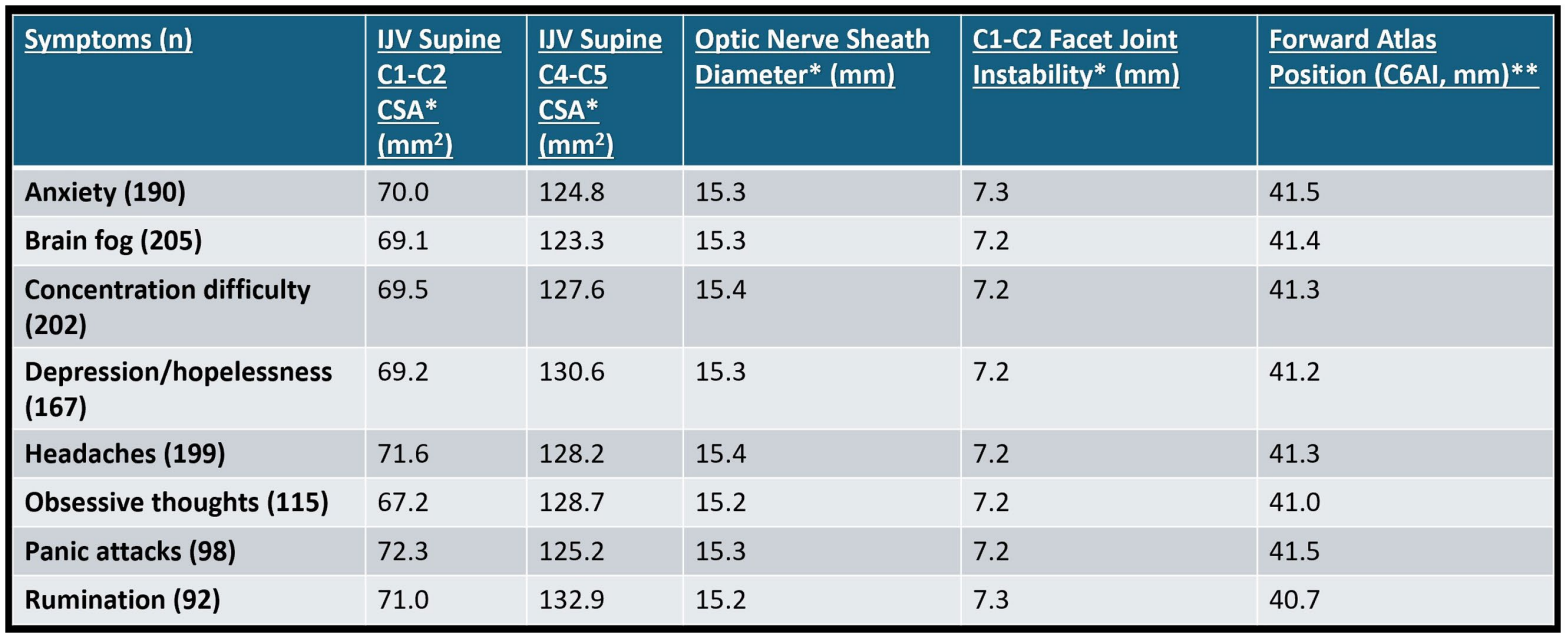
Summary of cervical structural analysis and ultrasound measurements of internal jugular veins (IJVs) and optic nerve sheath diameters (ONSDs) in 227 consecutive patients aged 20–50 who came to an outpatient neck center and had at least 1 of the 8 symptoms above, with no history of trauma or etiology of symptoms. * Mean bilateral total internal jugular vein cross-sectional areas (CSAs), ONSDs, and C1-C2 facet joint instability in patients with various brain/mental health symptoms. Normal for IV CSA >180 mm^2^, ONSD is <12.2 mm, and optimal C1 overhang on C2 in lateral flexion, open mouth is <2–4 mm.  C6A1 = C6-atlas interval, horizontal distance in the sagittal plane of the posterior inferior C6 vertebra to anterior atlas (optimal is <10 mm). Measures how far forward the atlas (C1) is in relation to lower cervical vertebra (C6).
